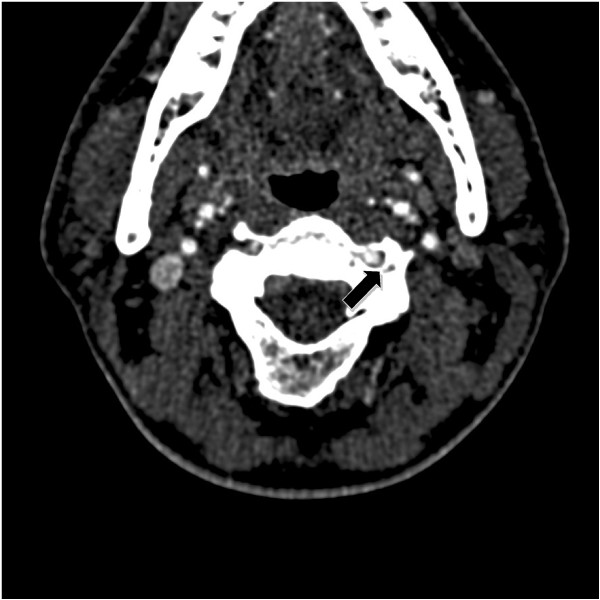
CT angiography showing a left vertebral artery dissection located at the C4 and C5 transverse foramina (arrow).
